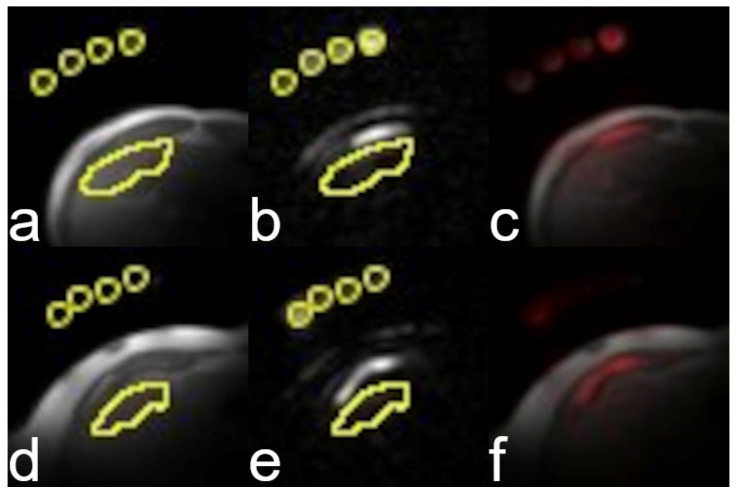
Representative MRI images from a healthy participant (a–c) and a participant with liver disease (d–f). Proton anatomical images (a,d) from each subject are presented for reference. Sodium signal intensity (b,e) from each subject overlaid in red on the proton image (c,f) to show sodium signal location. Small circular yellow regions-of-interest (ROIs) are the reference vials. The liver ROI for each subject is also outlined in yellow. The brightest sodium signal comes from the rectus abdominis muscle.
